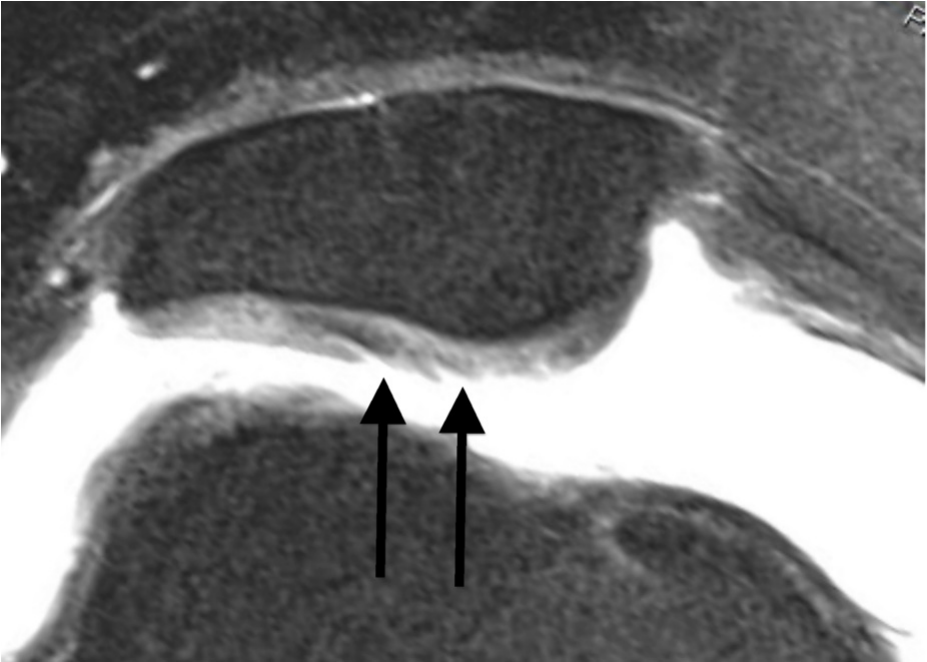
Axial PD-weighted TSE MRI of a 29-year-old male with a recurrent LPD. MRI shows a fibrillation, fissuring, or erosion composing < 50% of the cartilage thickness at the central dome and the lateral facet of the retropatellar articular surface (black arrow). This finding is defined as a grade 2 disorder.
